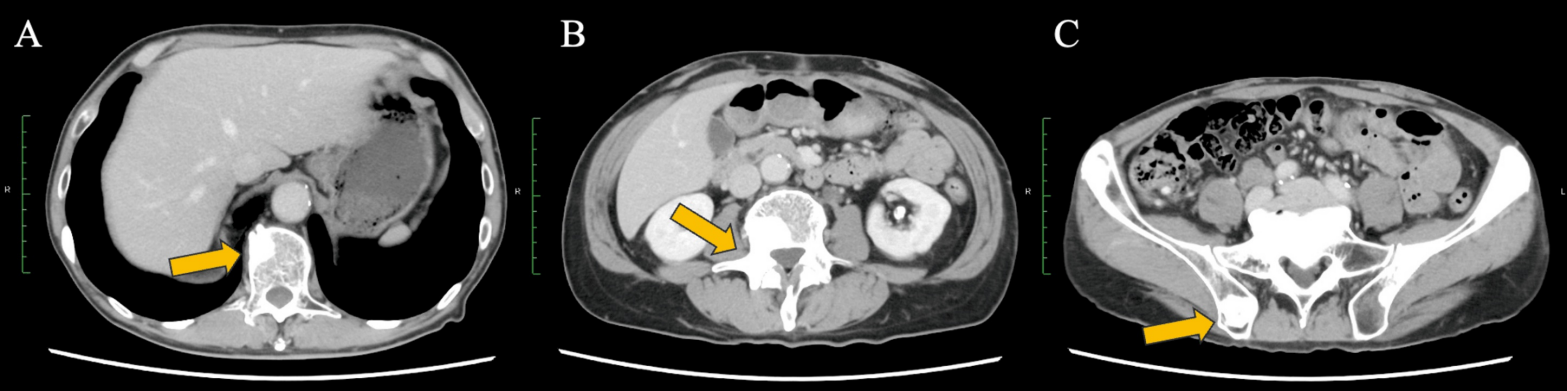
CT image obtained after treatment with enzalutamide plus degarelix (A, B, C): Post-treatment CT demonstrated persistent bone lesions without radiologic worsening (arrows) and no new sites of metastasis.
